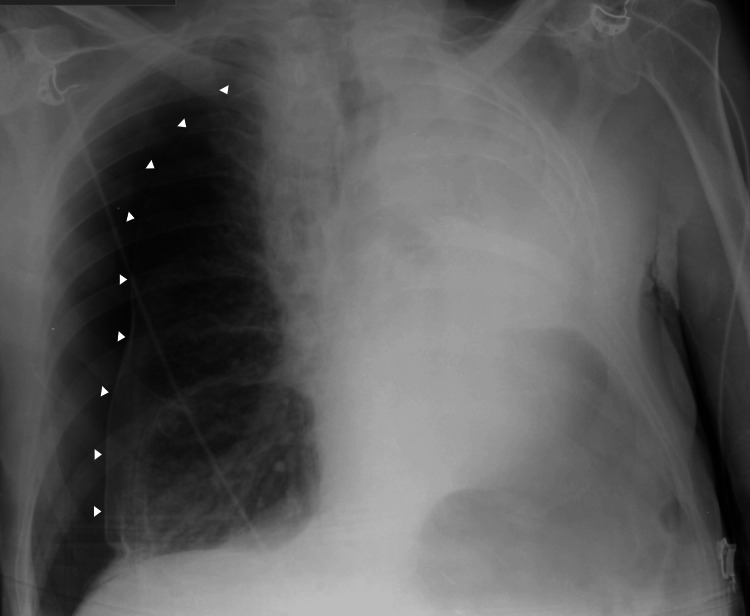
Recurrent right-sided pneumothorax. Chest radiograph obtained at readmission demonstrating a recurrent right-sided pneumothorax. Arrowheads delineate the visceral pleural line, consistent with lung collapse.
